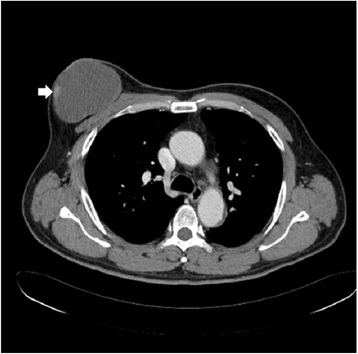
Chest CT shows a 9.3 × 6.7 cm, well-defined, thin-walled cystic mass in the right subareolar region with nodular enhancing lesions in the lateral wall, suggesting a benign soft tissue mass such as a large epidermal inclusion cyst
